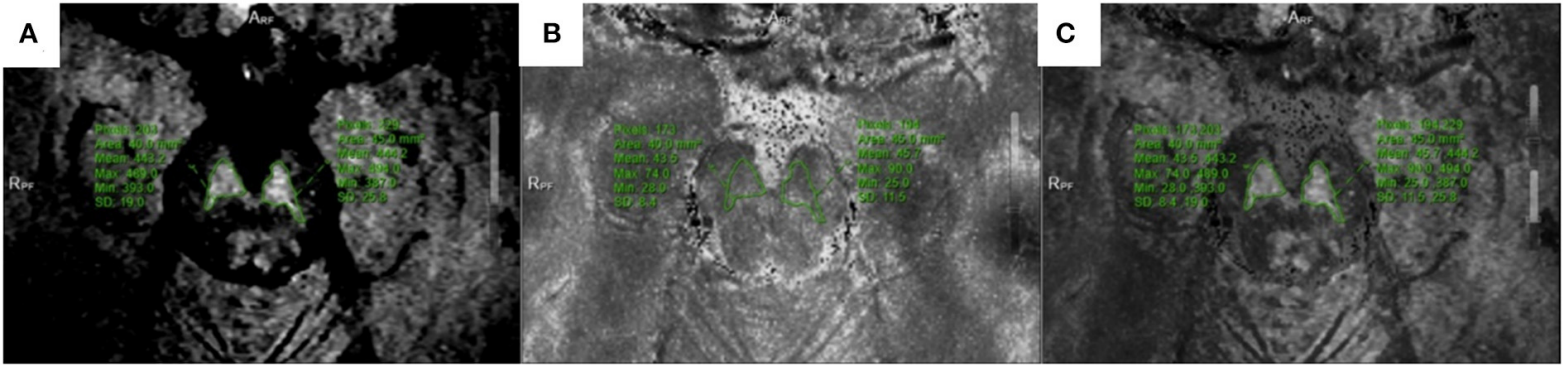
Located in the lower of SNc. (A) NM-MRI map; (B) T2*mapping; (C) Combine the two maps and obtain T2* value.
